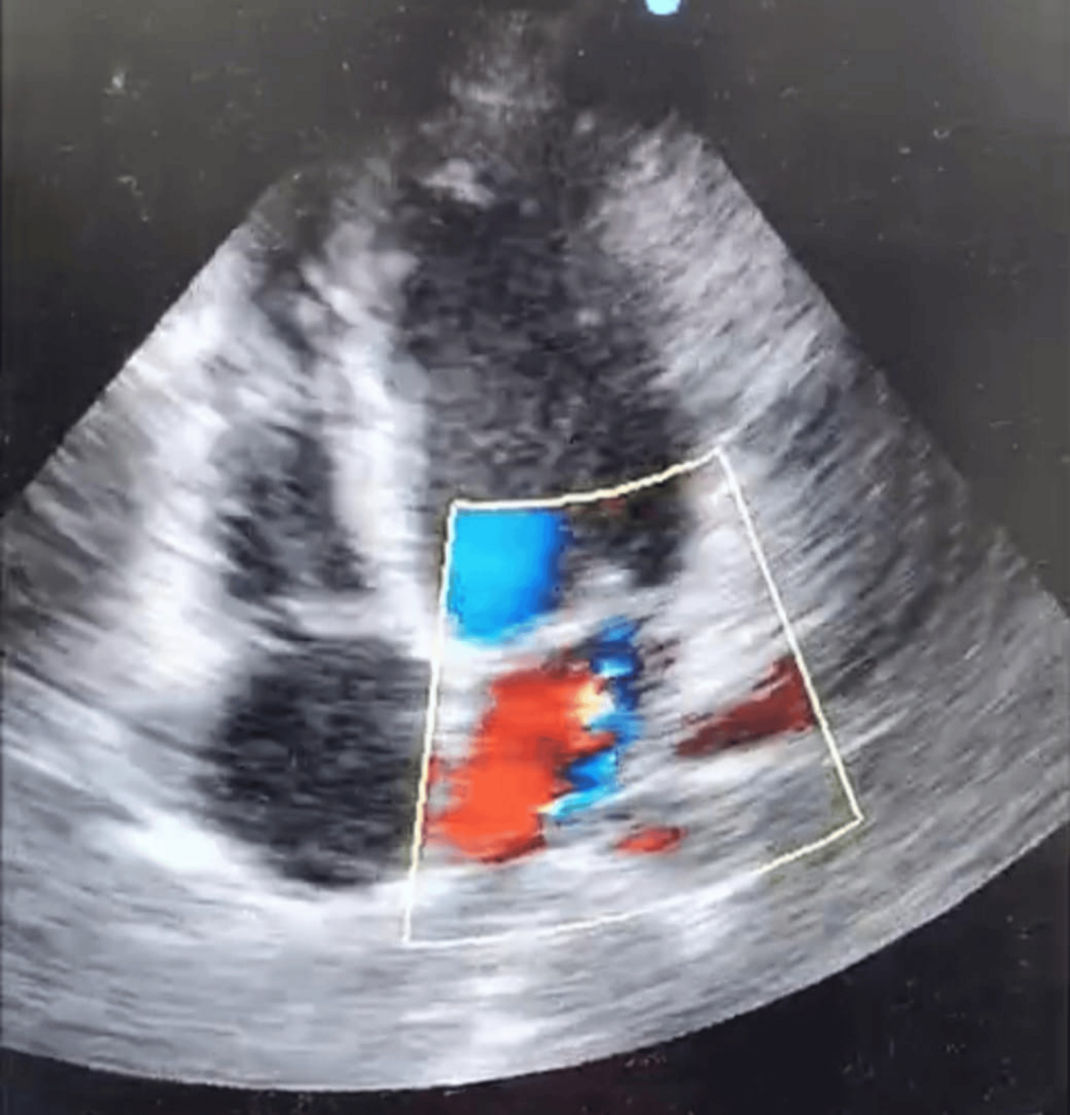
In the parasternal long axis (PLAX) echocardiographic view, the color Doppler demonstrates mitral regurgitation indicating rheumatic impression of carditis. The PLAX color Doppler view shows a significant turbulent (mosaic) flow jet across the mitral valve region. This is classic for mitral regurgitation, indicating blood is leaking from the left ventricle back into the left atrium during systole.
